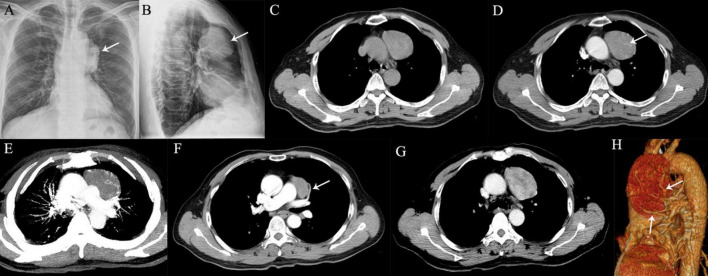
A 64-year-old man with SFTP. (A,B) Chest radiographs show a well-defined, homogeneous density opacity projecting the anterior left hilum (arrows). It was misdiagnosed as a mediastinal mass preoperatively. (C) Precontrast CT scans demonstrated an oval mass with well-delineated margin abutting the mediastinal surface. (D) Enlarged intratumoral vessels are seen in the arterial phase on post-contrast CT image (arrow). (E) The intratumoral vessels are depicted more clearer on MIP image. (F) A thick feeding artery arising from the left upper pulmonary artery is also detected (arrow). (G) Heterogeneous moderate to marked enhancement is detected in the venous phase. (H) Several feeding arteries arising from the left upper pulmonary artery to the tumor are showed on 3D VR image (arrows).
